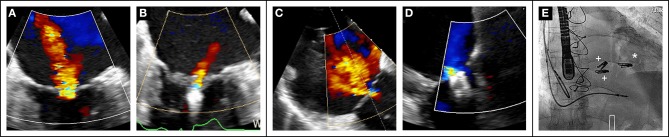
Case of combined mitral and tricuspid edge-to-edge repair in a 79-year old sympatomatic (NYHA III) male patient with prohibitive surgical risk (Euroscore II 21%). Baseline transesophageal assessment showed severe MR (A). After transseptal puncture, the mitral valve was treated first with the placement of one MitraClip XTR. with MR reduction to grade 1+ (B). Severe TR at baseline (C) Two MitraClip XTR were placed in the anteroseptal commissure with reduction of TR to mild (D). Final fluoroscopic result ((E), *MitraClip mitral, + MitraClips tricuspid).
